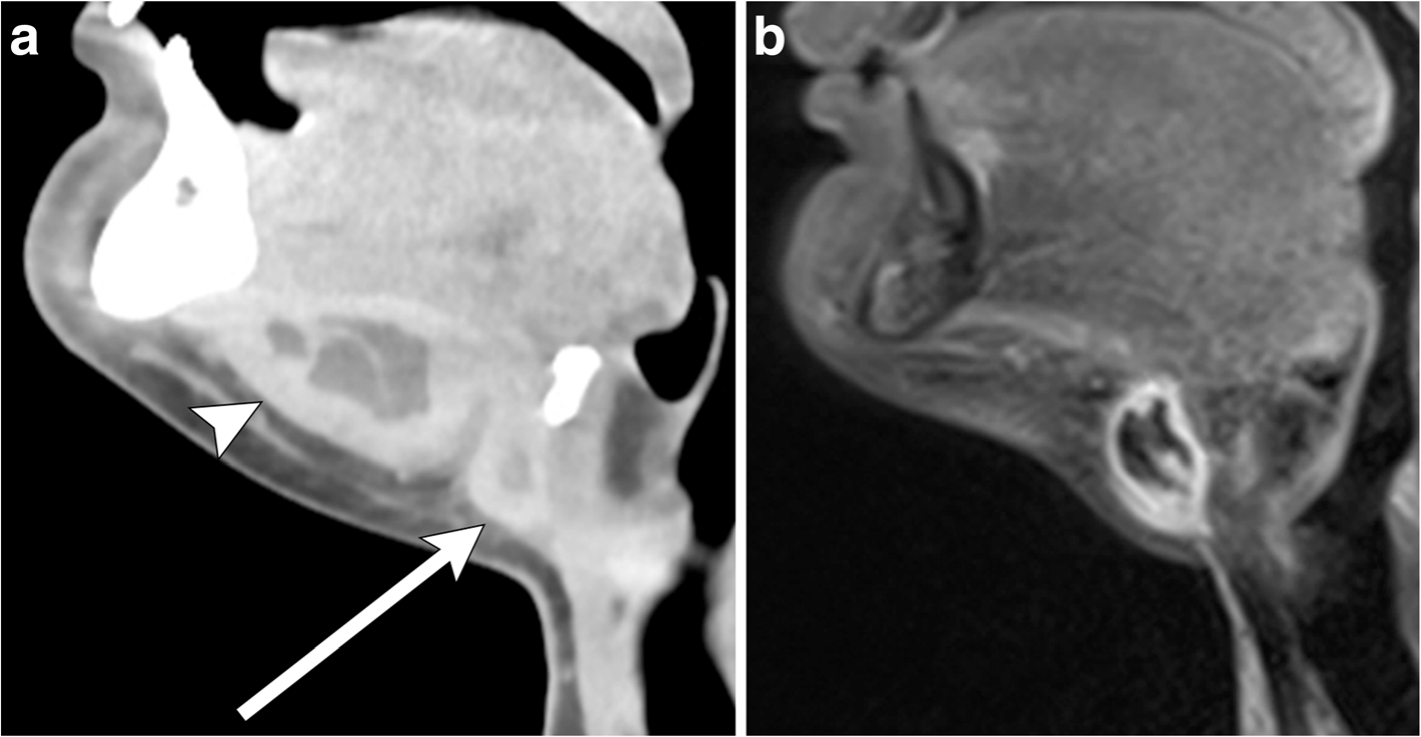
Infected thyroglossal duct cyst with abscess. a Sagittal contrast-enhanced CT image of the neck shows a thick-walled cyst adjacent to the hyoid bone (arrow). There is a communicating peripherally enhancing fluid collection along the floor of the mouth, consistent with abscess (arrowhead). Note stranding of the adjacent fat. b Sagittal post-contrast T1-weighted MR imaging of the neck demonstrates interval resolution of the abscess and underlying thyroglossal duct cyst with residual inflammatory changes. Note the relative increased thickness of the wall of the infected thyroglossal duct cyst compared to one that is not infected (see Fig. 2)
